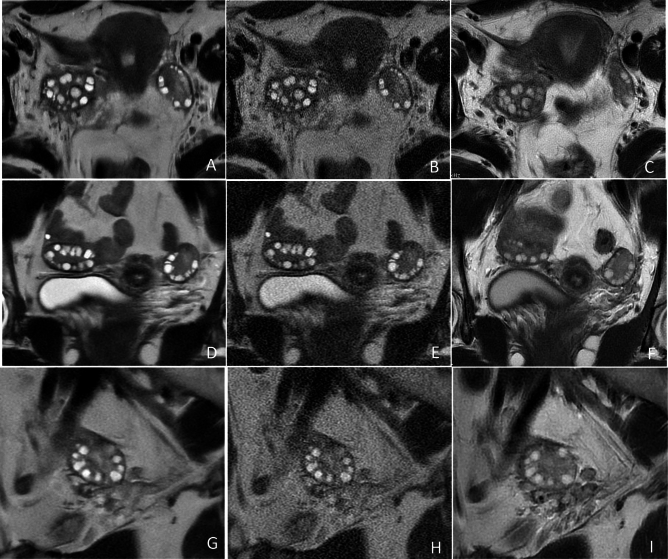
Representative T2-weighted MR images of the ovaries in a 17-year-old adolescent girl with PCOS. The SSFSE-DL images (A–G) clearly depict bilaterally enlarged ovaries and an increased number of peripheral follicles with minimal noise and blurring artifacts, providing the best conspicuity of follicles. The use of AIR Recon DL enhances the contrast between follicles and the surrounding ovarian stroma. The conspicuity of follicles in the SSFSE-C images (B, E, H) is mainly affected by noise, while that in the PROPELLER images (C, F, I) is primarily impaired by motion-related blurring artifacts.
